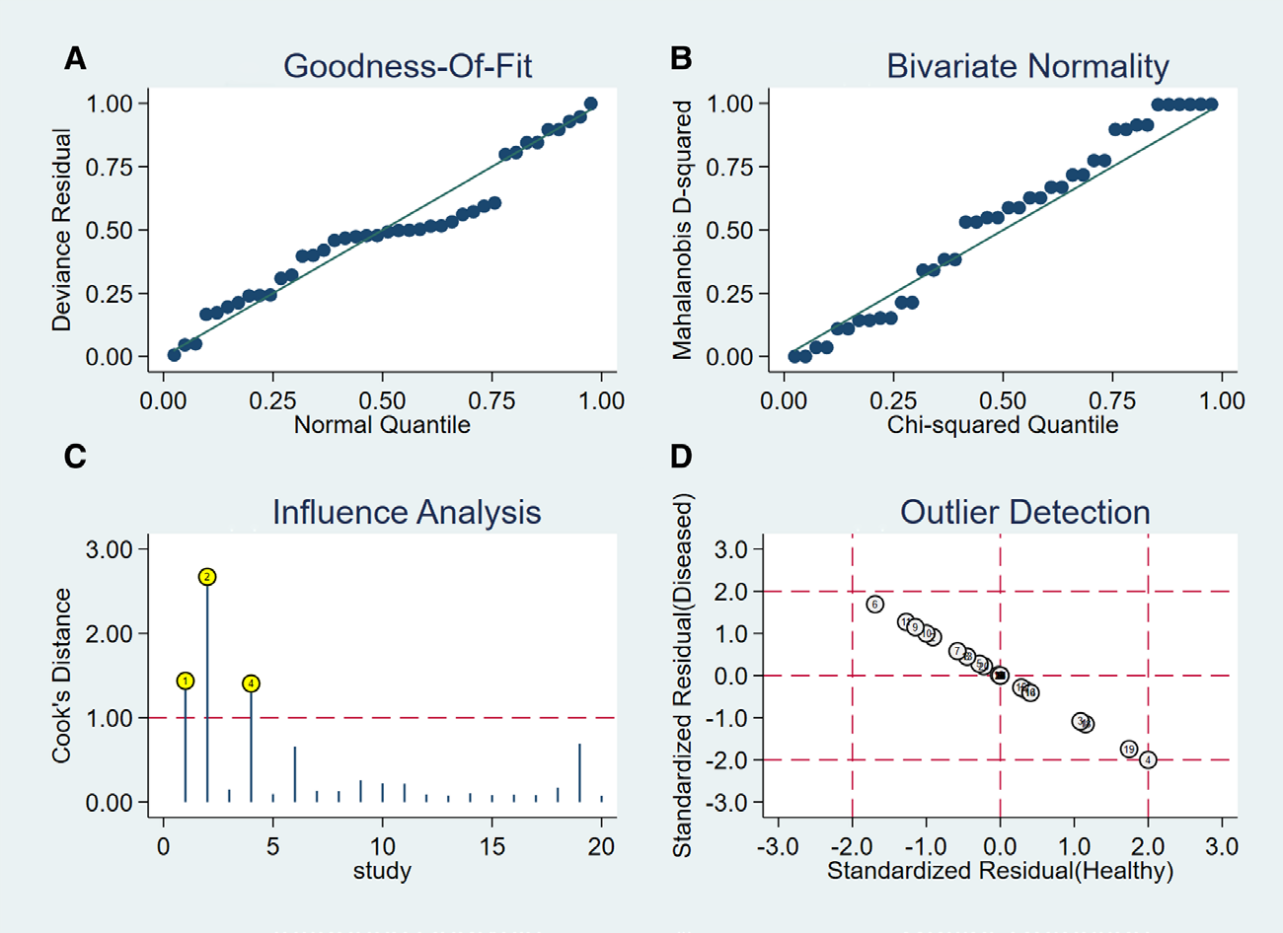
The sensitivity analysis of MRI. MRI = magnetic resonance imaging.
